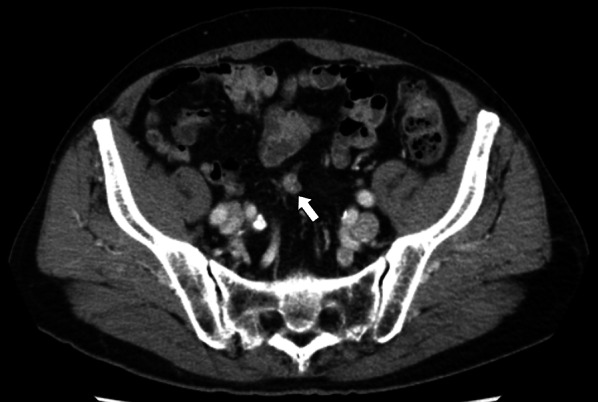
CT showed a nodular lesion in the sigmoid mesentery
